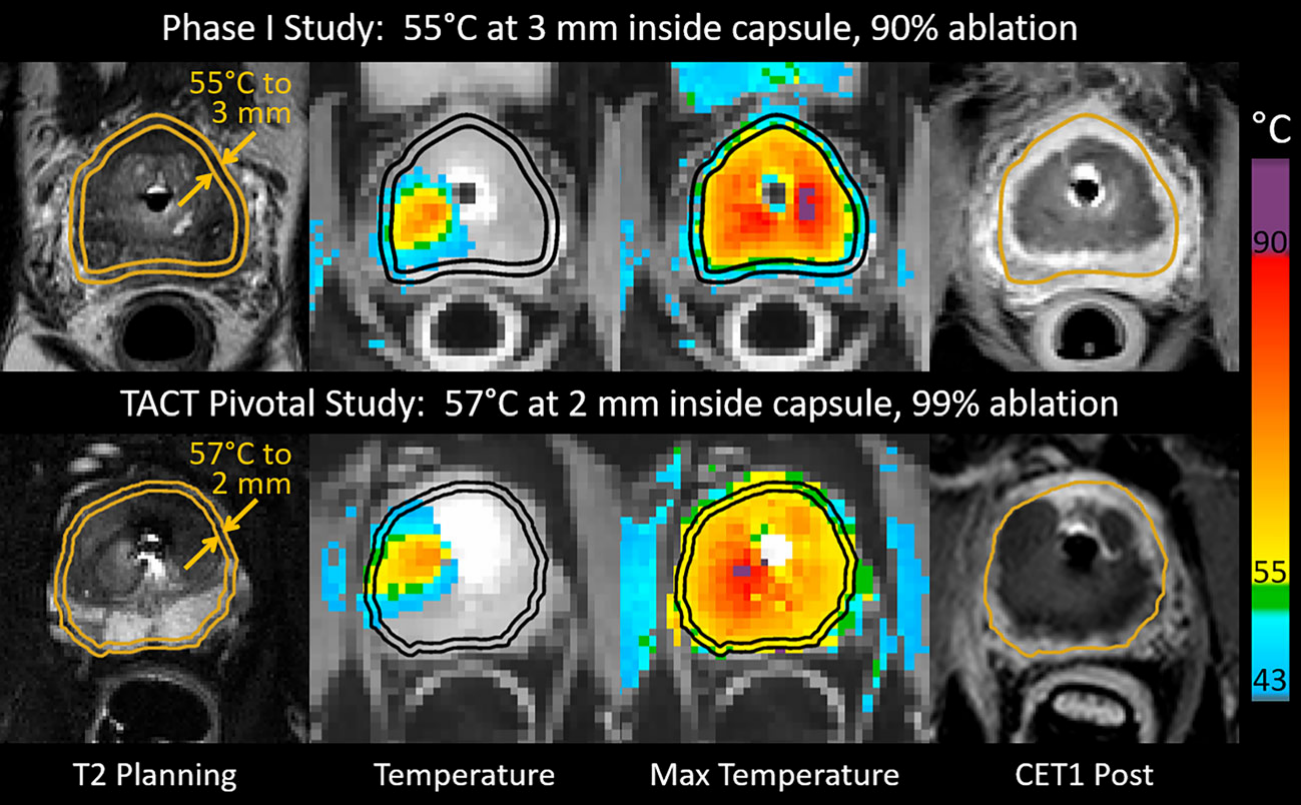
Intraoperative images from patients who underwent MRI-guided transurethral ultrasound ablation (TULSA) using conservative treatment parameters in the Phase I study (top), and intensified parameters designed to achieve whole-gland ablation in the pivotal study (bottom). Transverse images at one location in the midgland for each patient depict interoperative treatment planning on T2-weighted images, real-time MRI temperature images used to control treatment, maximum temperature projections used to assess ablation coverage during treatment, and post-treatment contrast-enhanced T1-weighted images (CET1) demonstrating greater ablation extent in the example from the pivotal study (bottom).
